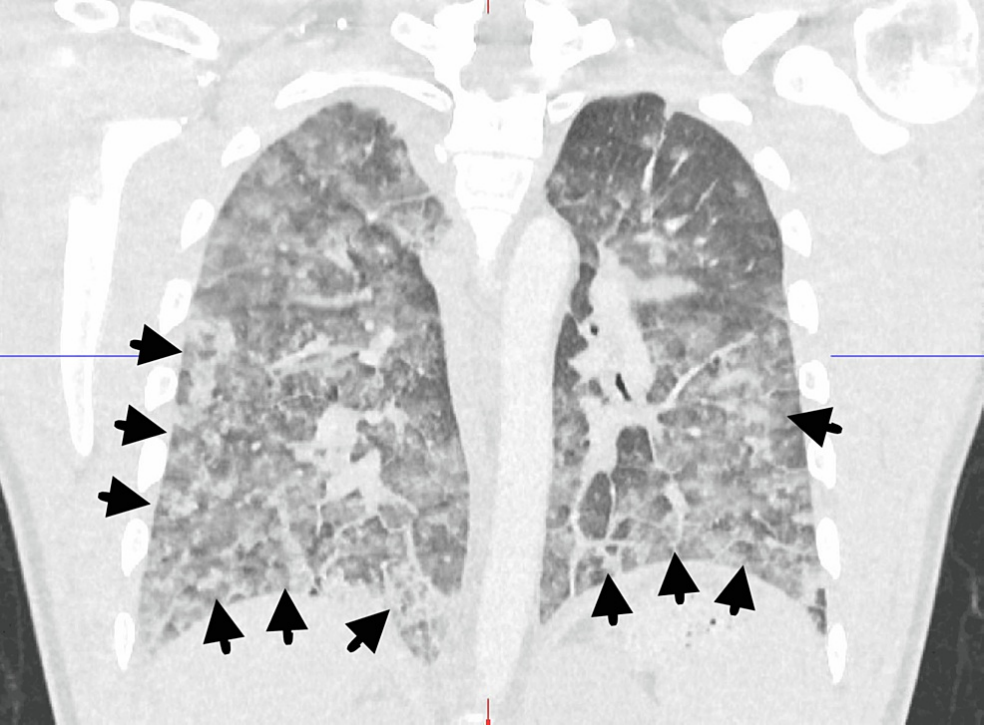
CT chest with contrast on initial presentation showing patchy consolidations and ground glass opacities in both lungs with basilar predominance denoted by black arrows
